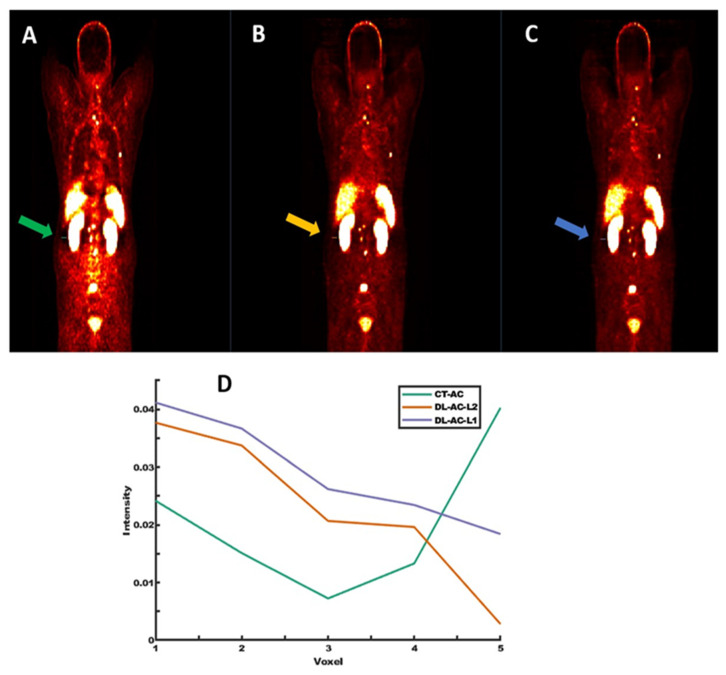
Representative images from (A) PET CT-AC with halo artifact, (B) PET DL-AC (L2 loss), and (C) PET DL-AC (L1 loss). (D) shows horizontal signal intensity profiles across the halo region, demonstrating better artifact suppression with DL-based methods (Green, orange, and purple lines correspond to the line profiles drawn on PET-CT, PET-DL-L2 loss, and PET-DL-L1 loss, respectively).
